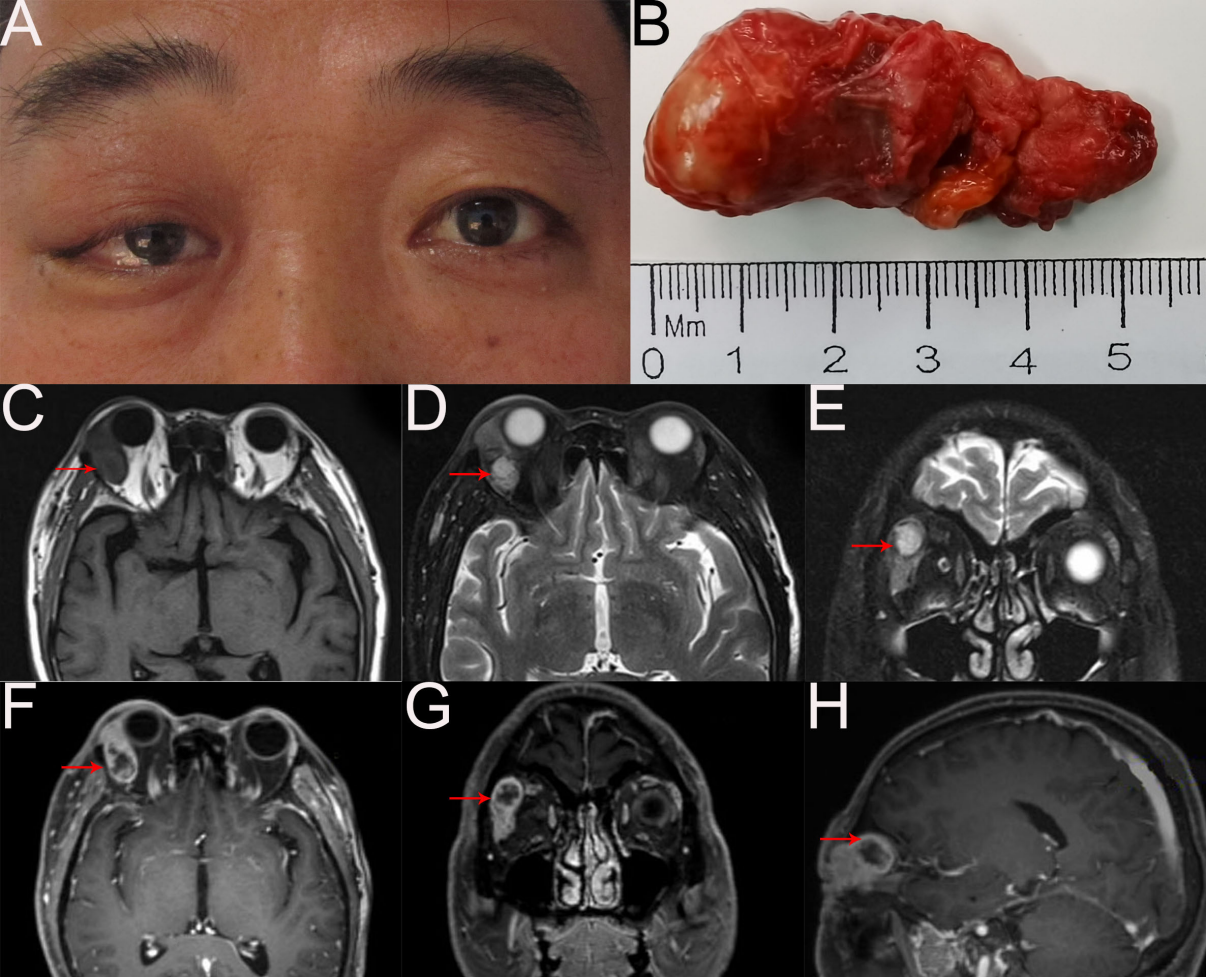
(A) External image of the patient. (B) The mass was dissected under the table and was approximately 5.5cm x 2.3cm x 1cm in size. (C–H) Orbital MRI scan revealed right lacrimal gland was enlarged obviously and an analogous round mixed-signal (2.3cm×2.0cm×1.5cm) shadow in the right lateral posterior superior orbit with heterogeneous enhancement. T1-weighted imaging without contract enhancement and fat suppression (C). T2-weighted imaging with contract enhancement and fat suppression (D) and (E). T1-weighted imaging with contract enhancement and fat suppression (F-H).
